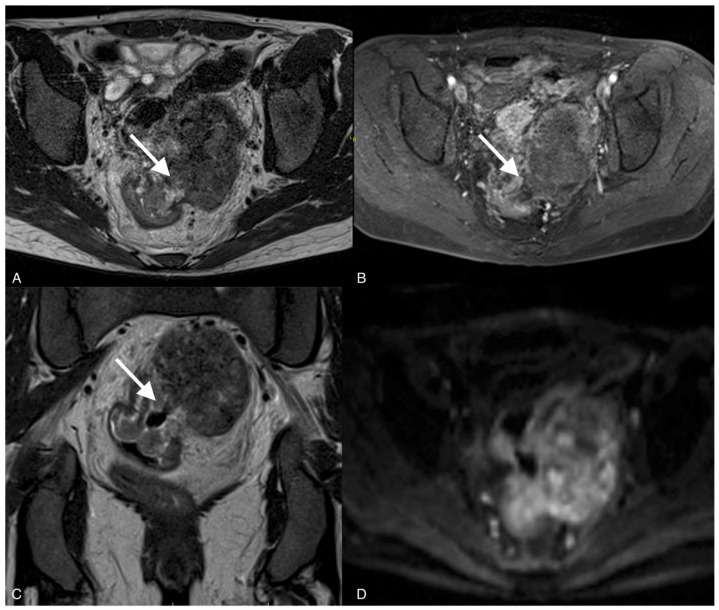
Images of a 37-year-old woman with a recurrent tumor of high-grade serous ovarian cancer with fistula between tumor and sigmoid colon. MRI T2-weighted images obtained in axial plane (A), coronal plane (B), and T1 post-contrast (C) show a large solid/cystic tumor and the fistula (arrows) between tumor and sigmoid colon. DWI (b1200) shows restricted diffusion in the tumor but not in the fistula (D).
